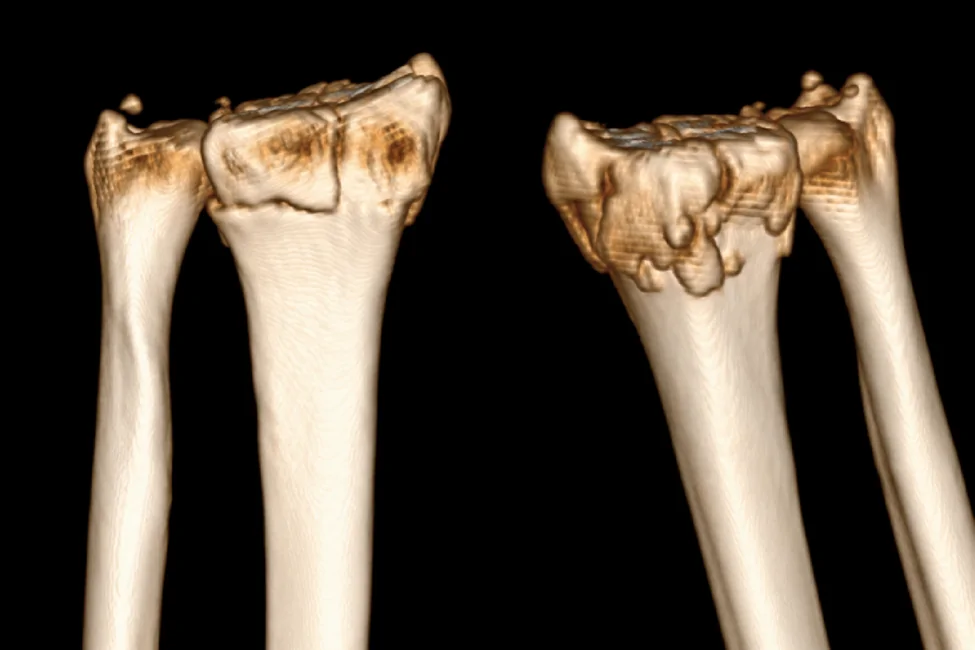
Representative 3D CT reconstruction images selected from the post-reduction GIF sequence of an AO type C distal radius fracture, showing the spatial configuration of the fracture fragments after reduction
